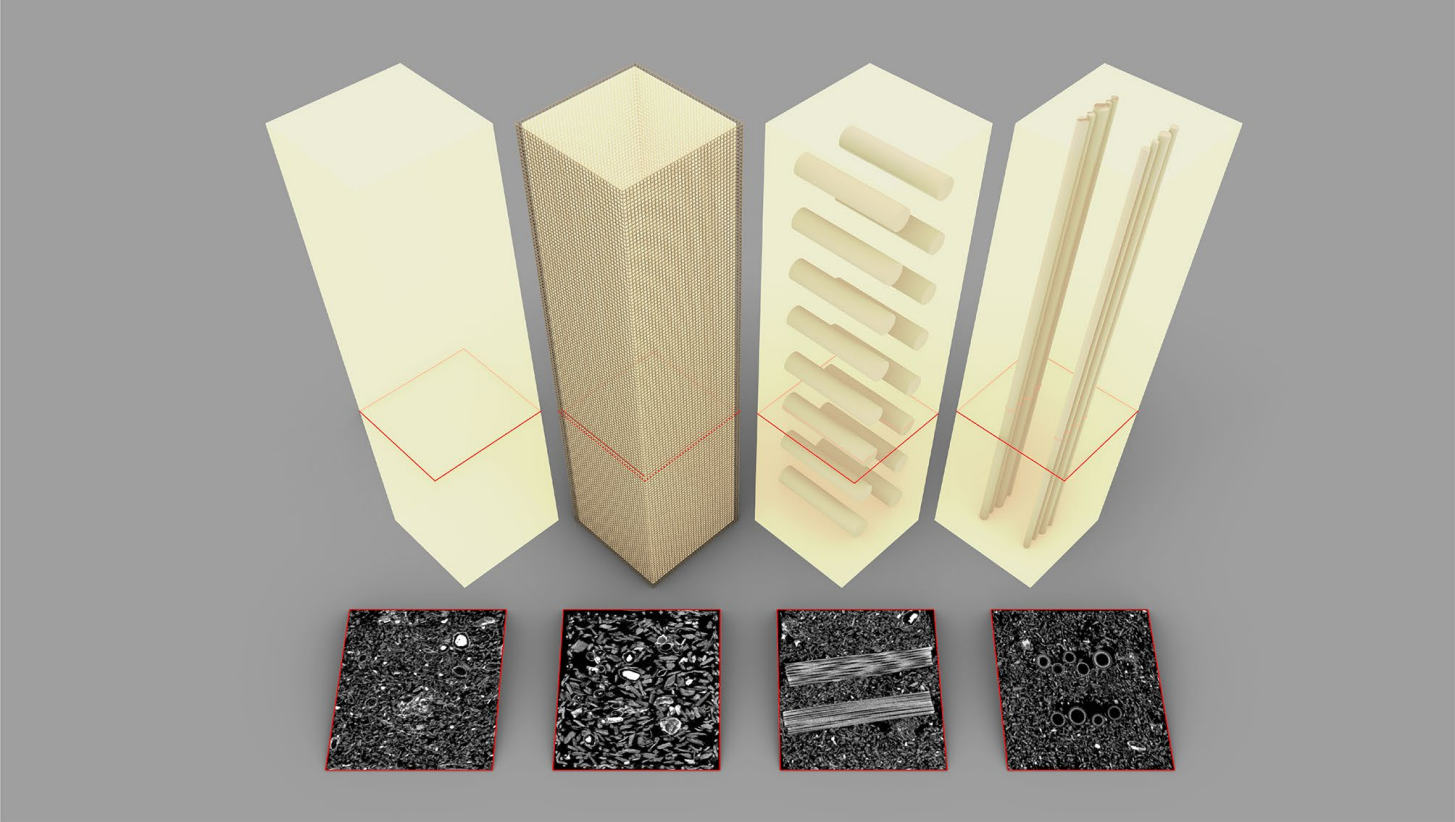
Fibre placement strategies and their sectional CT scan (left to right): control (BS), jacketing coaxial to load (BM_H), fibres perpendicular to load (BS_R), fibres coaxial to load (BS_V).
